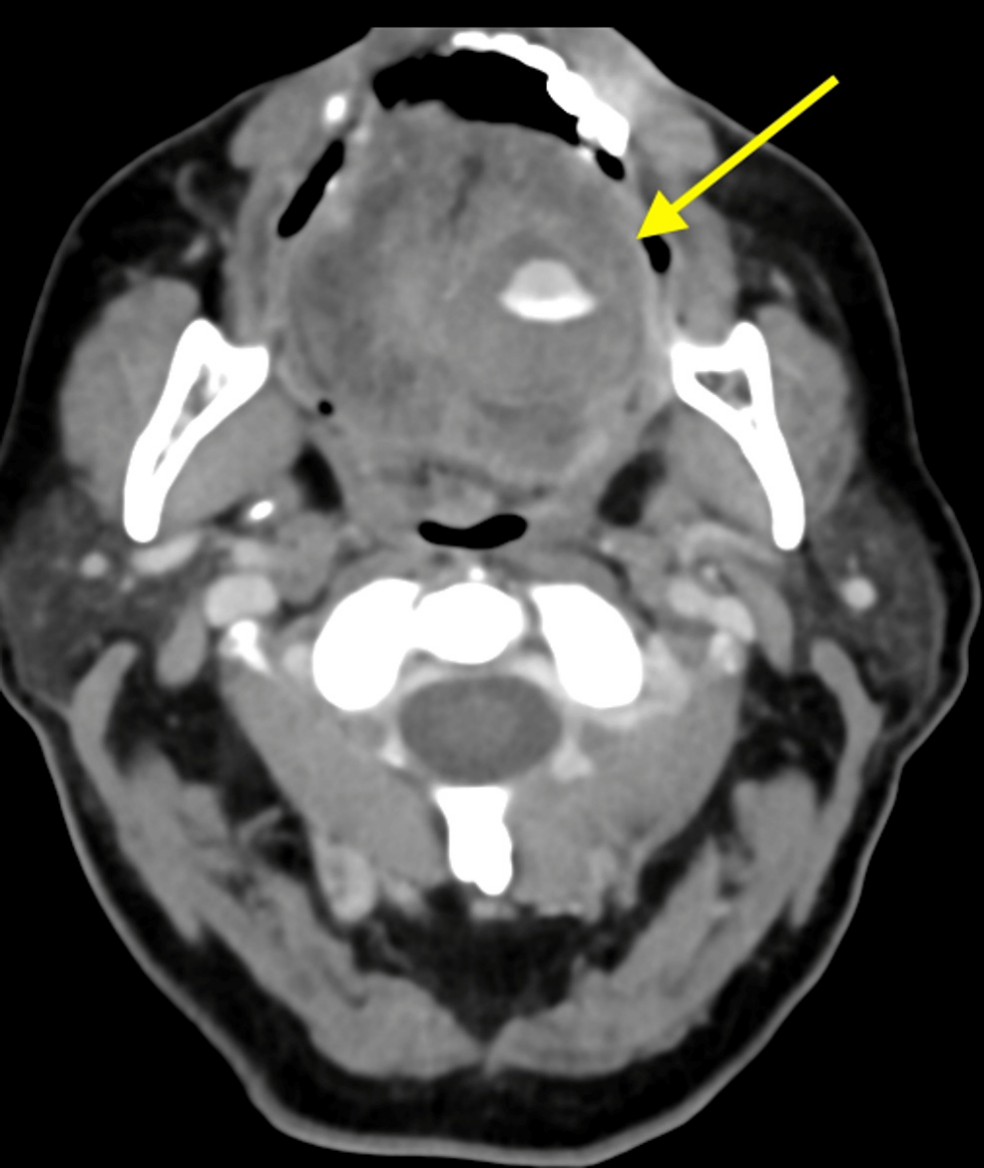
Axial head non-contrast computed tomography demonstrating ventral tongue lesion (arrow). The lesion has a similar appearance to other vascular structures in the same viewing window (e.g., carotid arteries and internal jugular veins).
